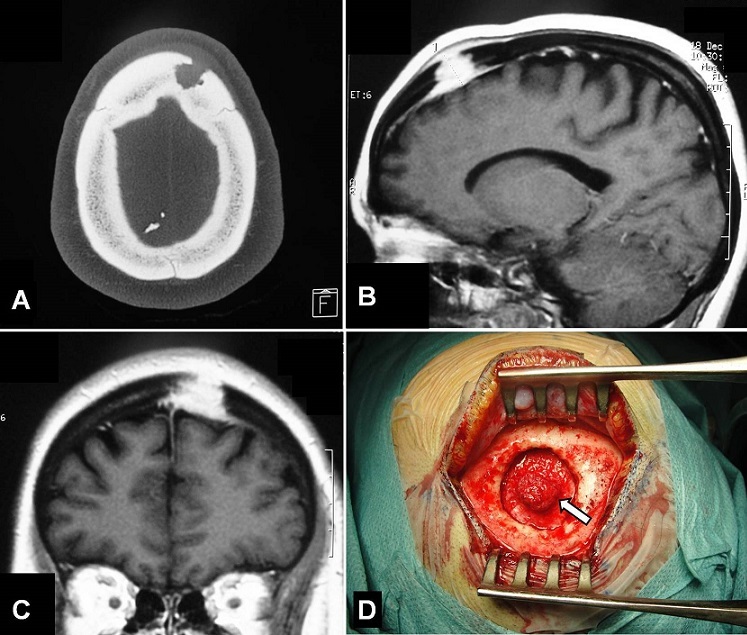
A) Axial CT-scan (bony window) revealing the left frontal bone defect; B and C) Sagittal and coronal T1-weighted MRI after gadolinium injection showing homogeneous enhancement of the lesion which was extradural and intraosseous with extra cranial and intracranial extension; D) Operative view of the tumor (arrow) after removing of the surrounding bone
